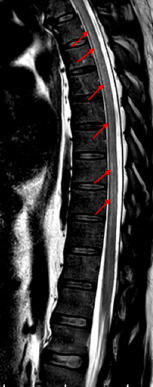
Sagittal *T*2 weighted image MRI shows slight hyperintensities in the ventral aspect of the upper and mid-thoracic cord (arrows).
